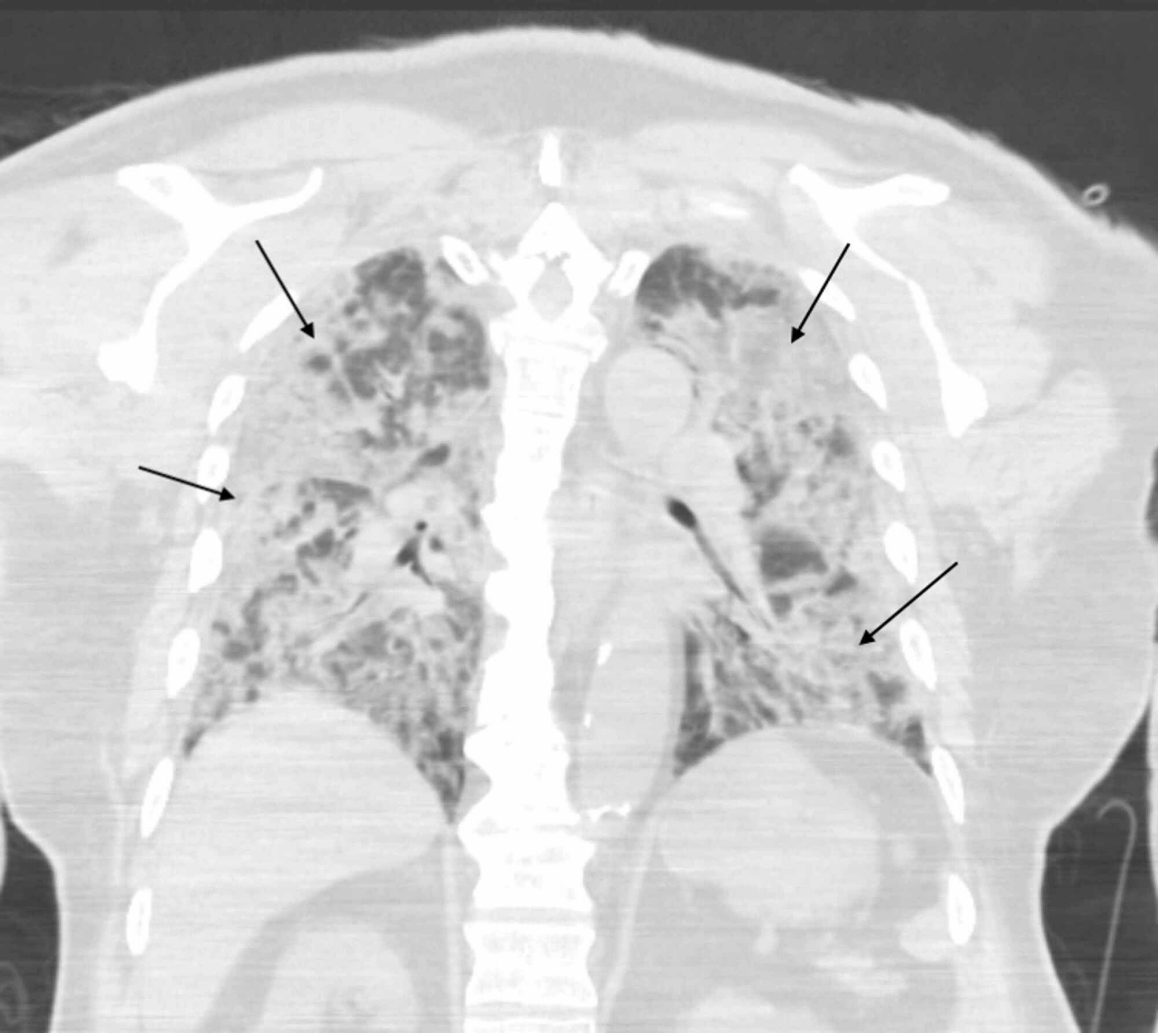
Chest CT scan. Bilateral consolidative infiltrates " arrows " on a follow up CT chest 48 hours after admission
